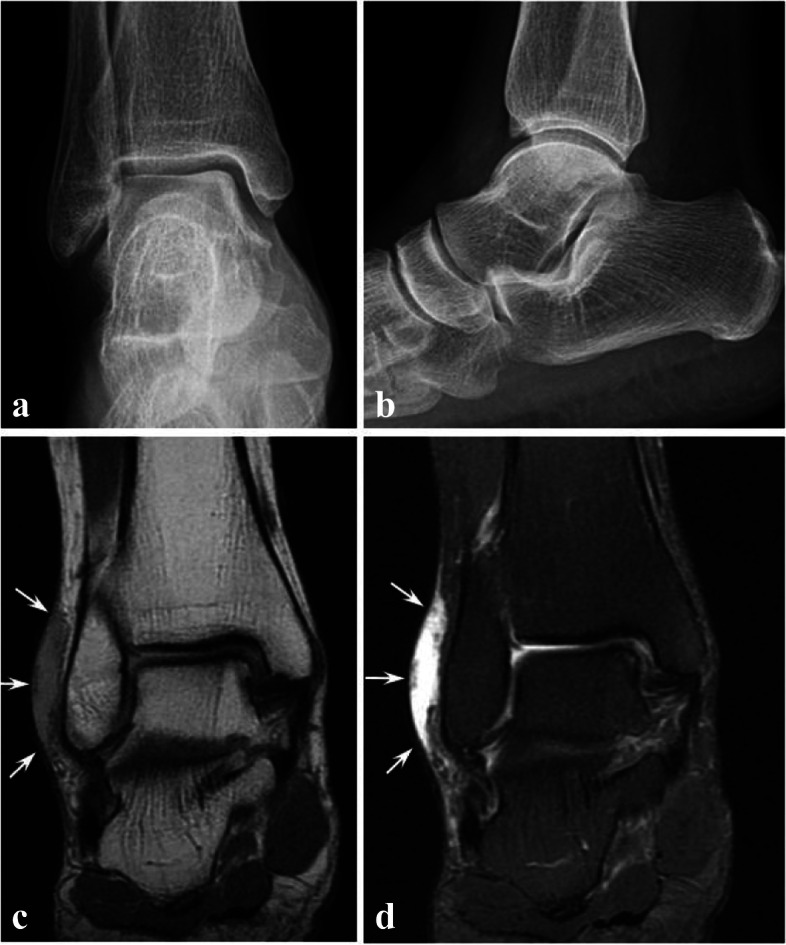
Initial plain radiographs and MRI from outside hospital. Plain radiographs (a, b) showing no osteolytic lesions around ankle joint. Coronal view of Proton density fat suppression image (c) and T2-weighted fat suppression image (d) showing lateral malleolar bursitis (white arrows)
